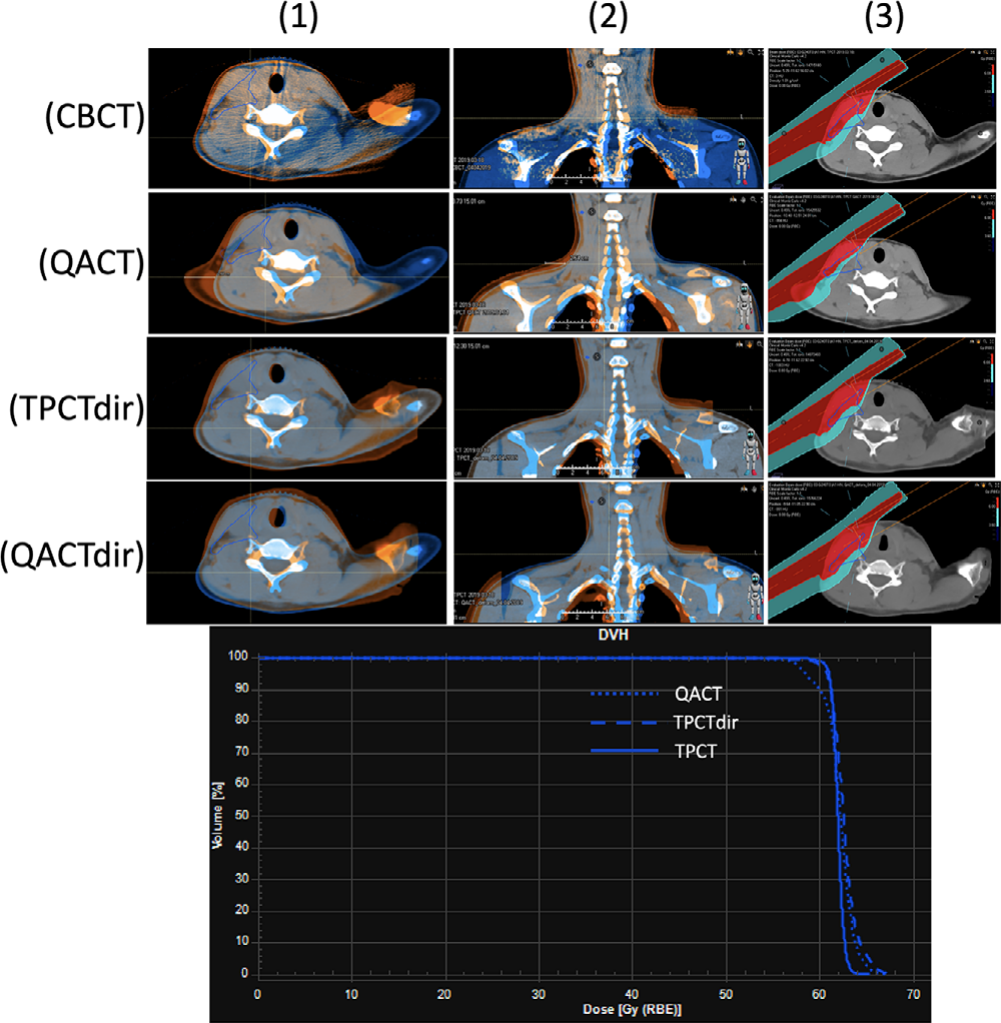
From top to the bottom are the image registration in axial (column 1), coronal (column 2), and their corresponding evaluation dose (column 3) from the posterior beam for the CBCT (row 1), QACT (row 2), TPCTdir (row 3), and QACTdir (row 4) with respect to the TPCT. The first row is from the initial nominal plan on the TPCT. The QACT shows a 2.64‐cm tissue difference at the neck region (second row) due to its inconsistent setup with the TPCT, while the CBCT, TPCTdir, and QACTdir match the TPCT better on the right (target) side. The dose volume histogram (DVH) plot (bottom) shows the incorrect node coverage reduction in the QACT (dotted blue line), correct evaluation in the TPCTdir (dashed line), and the initial coverage in the original plan (solid line). CBCT, cone‐beam CT; QACT, quality assurance CT; TPCT, treatment planning CT; TPCTdir, deformed TPCT to CBCT; QACTdir, deformed QACT to CBCT
